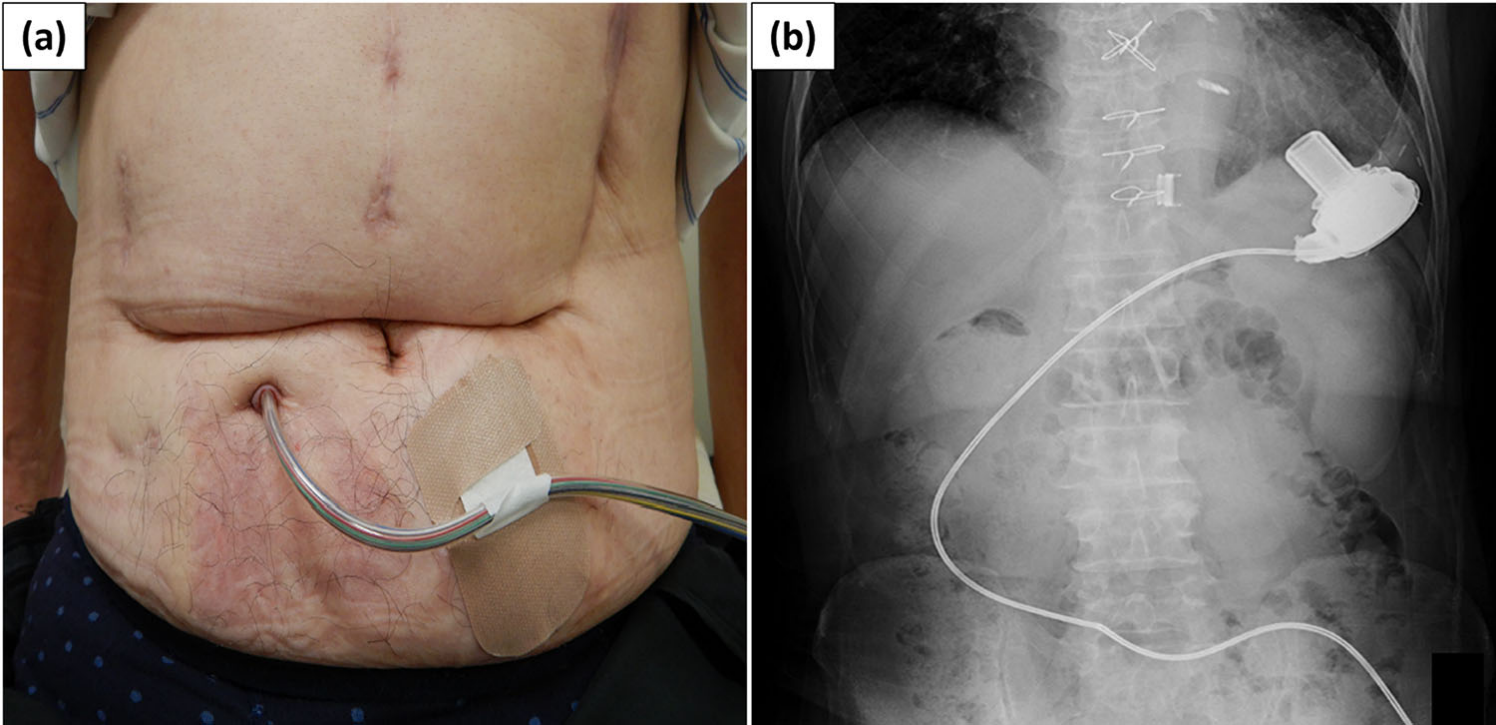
(a, b) The driveline is guided out of the body through the right hypochondrium, and radiographs show a HeartWare ventricular assist device positioned in the cardiac apex.
